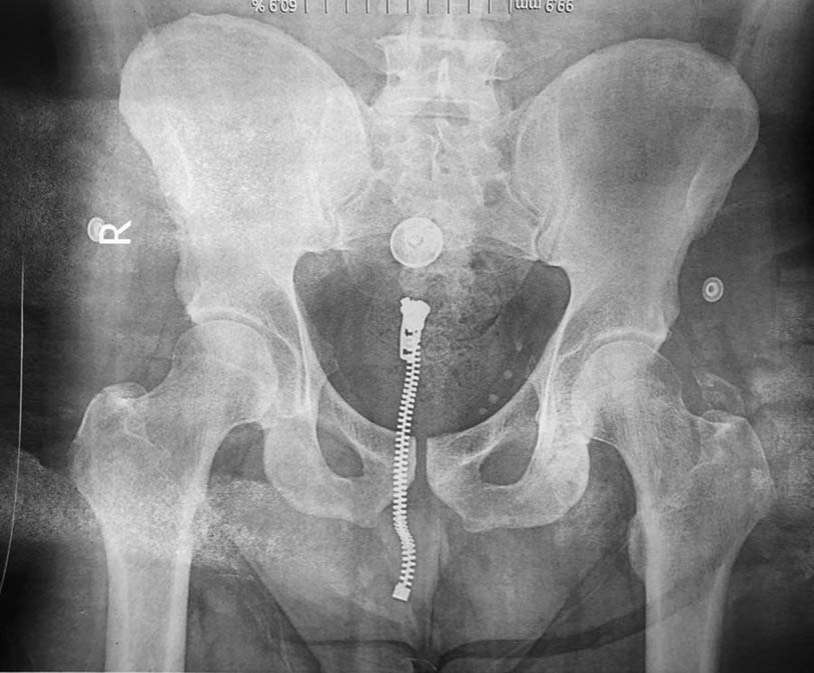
A post-reduction anterior-posterior pelvic view X-ray. The femoral head is seen in the acetabulum.
